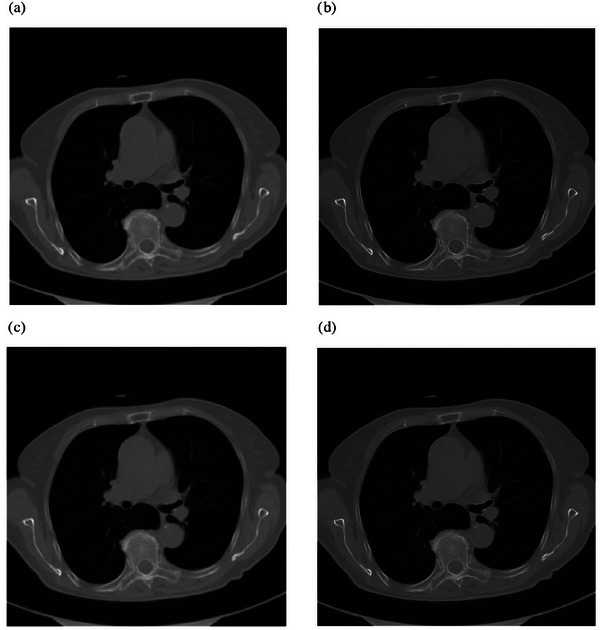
Clinical chest CT images after reconstruction.
